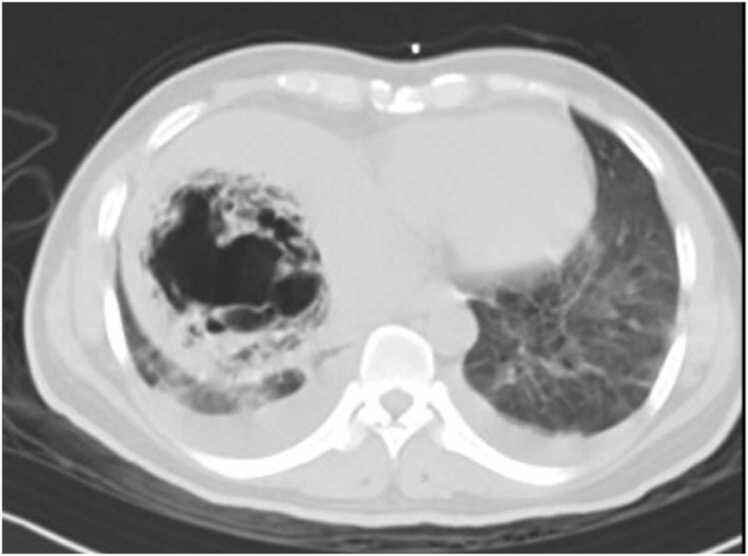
Abdominal CT demonstrates an abscess 8 cm in diameter in the right liver lobe and air tracking along the hepatic vein.
